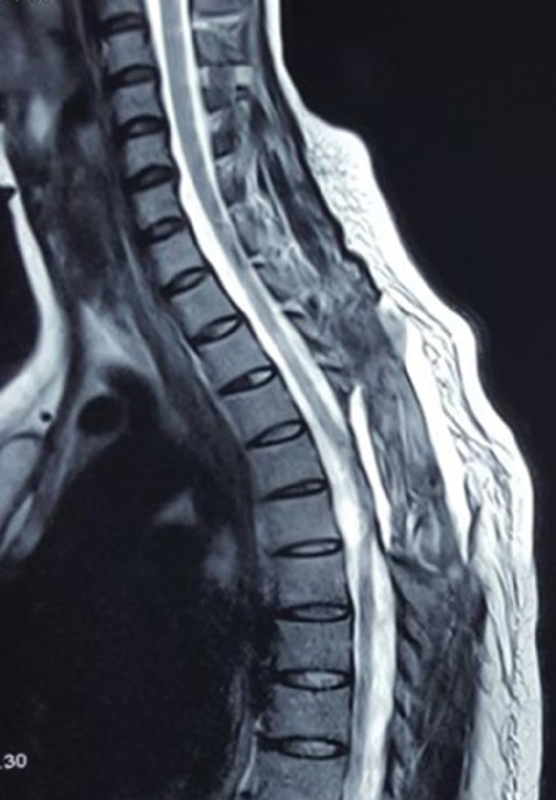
Postoperative T2-weighted MRI showing complete tumor removal. MRI, magnetic resonance imaging.
